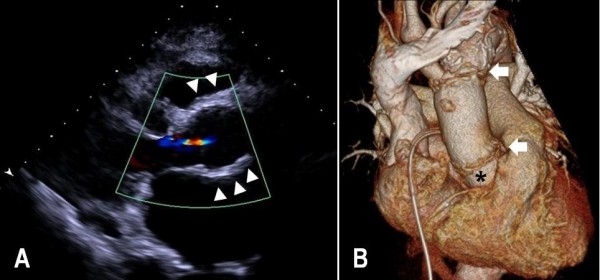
Post-operative images. (A) Post-operative transthoracic echocardiographic image with color flow mapping from the parasternal long-axis vies in patient 1. The geometry of the aortic root reinforced by wrapping a Dacron graft (Arrowheads) is well restored and maintained as a bulging morphology. And well-coaptating aortic valve cusps with trivial aortic regurgitation are also noted. (B) Post-operative three-dimensional volume rendering of contrast-enhanced CT scans in patient 1. It reveals that the ascending aorta was replaced with a prosthetic graft and the aortic root was reinforced by wrapping a Dacron graft (asterisk). Also proximal and distal anastomotic lines (arrow) were visible.
